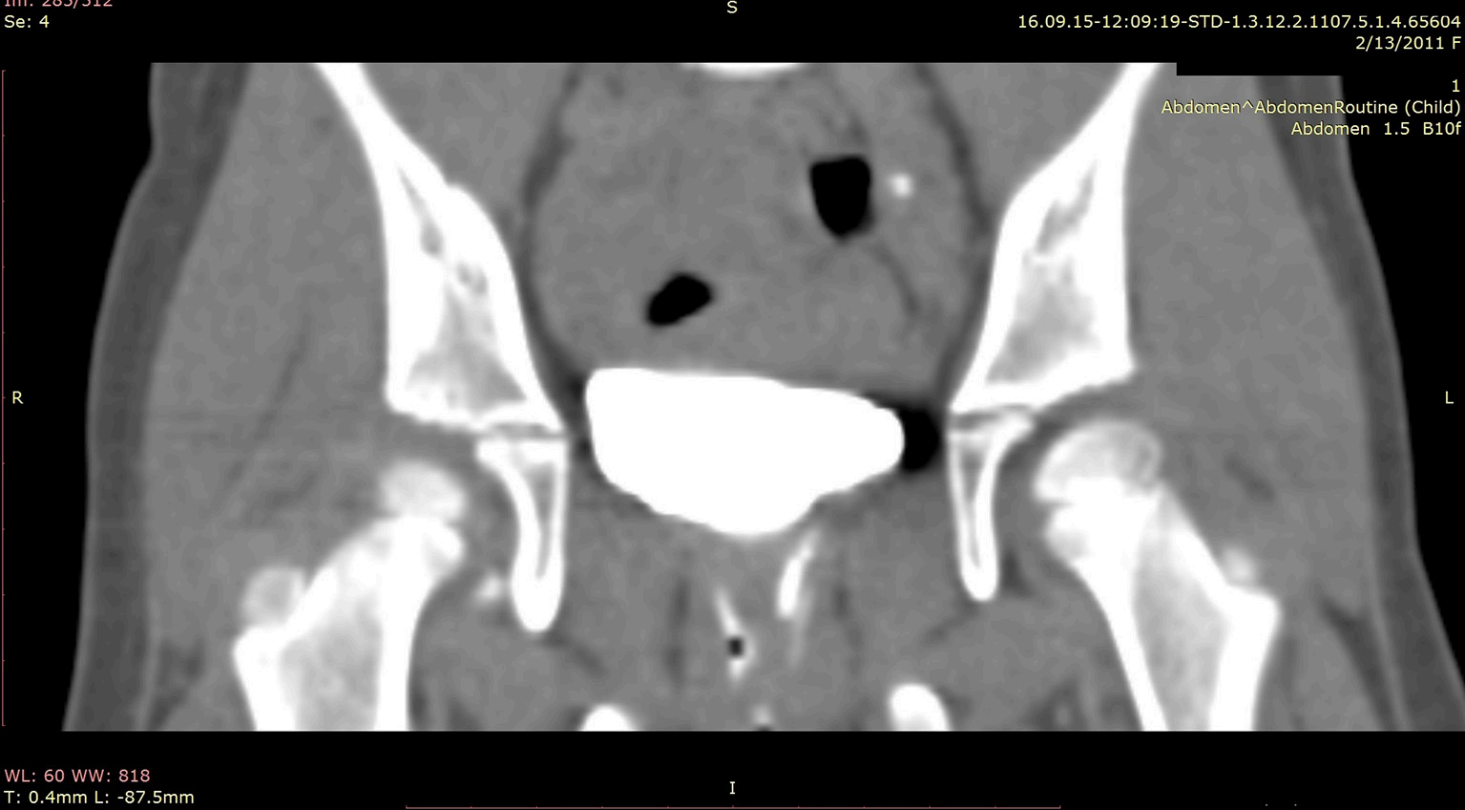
CT urography coronal view—double ectopic left ureter abuts the vagina under the bladder.
